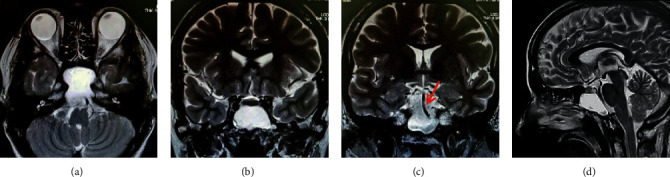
Noncontrast magnetic resonance T2-weighted imaging of the paranasal cavity at the initial diagnosis. (a) Axial, (b, c) coronal, and (d) sagittal views. The basilar artery located adjacent to the cyst (c) is indicated with the red arrow, and the cyst located adjacent to the brainstem (d) is indicated with the white arrow; however, the signal intensity of the lesion's contents is determined to be different from that of cerebrospinal fluid, therefore suggesting a primary cyst.
